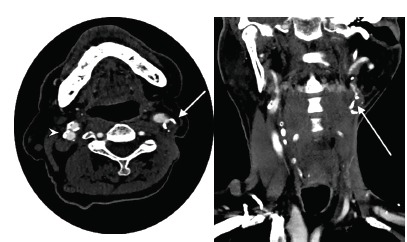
Computed tomography angiography of the head and neck in coronal (right) and axial (left) views demonstrating complete occlusion of the left internal carotid artery (ICA) (arrows) and critical narrowing of the right ICA (arrowhead), in a patient with right central retinal artery occlusion.
